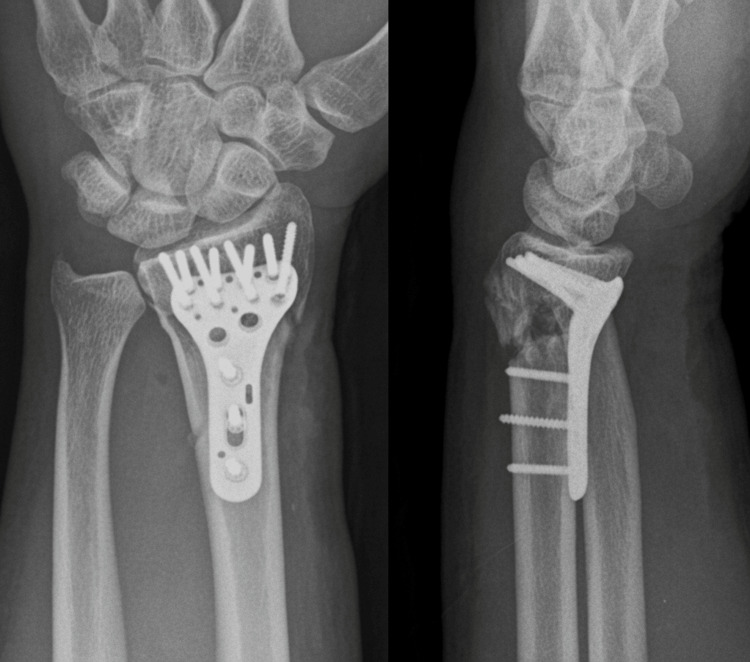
Postoperative radiographs after volar plate fixation Postoperative plain radiographs demonstrating fixation with a volar locking plate and restoration of the appropriate alignment of the distal radius
